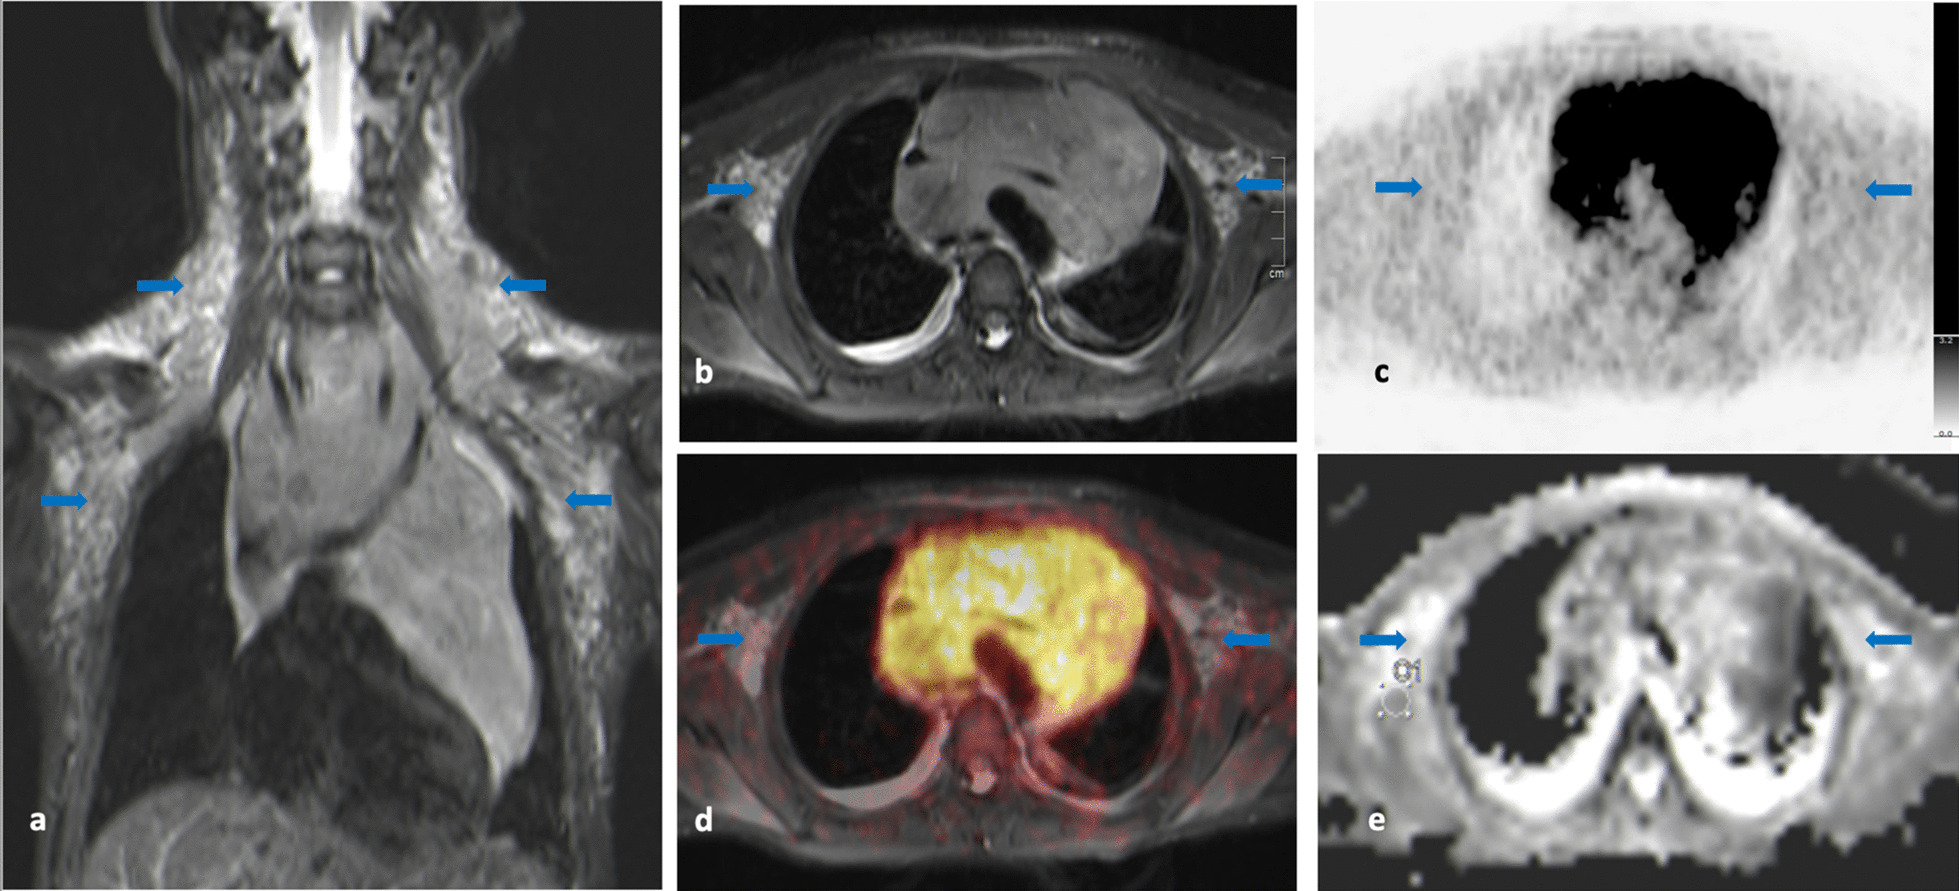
a–e Neck, axillae and chest on T2-TIRM image in coronal view (a); transversal view of axillae and chest on T2-TIRM (b), [^18^F]-FDG-PET (c), [^18^F]-FDG-PET/MR (d) and DWI at b-value of 800 (e): Lymphoma masses on both sides of the neck, with multiple, small lymph nodes extend caudally and filling both axillae (blue arrows) (a, b). In addition, diffuse lymphoma involvement of the thymus can be detected (a). Axillary lymph nodes exhibit physiological glucose uptake (SUV_max_ right: 1.17; SUV_max_ left: 1.25) (c, d) without restricted diffusion (ADC right: 1693 × 10^–6^ mm^2^/s; ADC left: 1746 × 10^–6^ mm^2^/s) (e)
